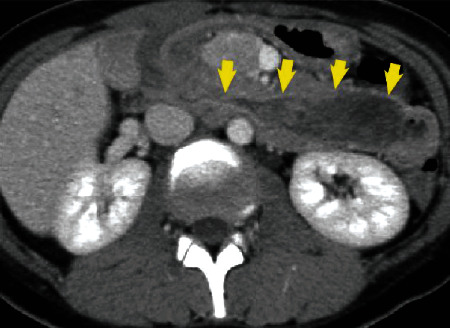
Abdominal CT showing a 7 cm mass extending from the bulb to the descending duodenum, with intussusception. The intrahepatic bile duct is not dilated.
